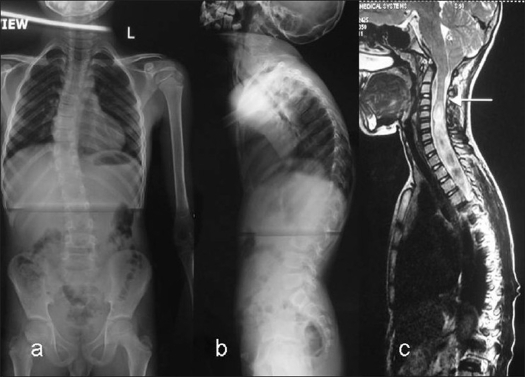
A 13 year old girl presented with a recent onset right thoracic scoliosis. Clinical examination was normal. (a, b) The whole spine X-ray showed a minimal coronal plane deformity, however there was a thoracic apical kyphosis. (c) The MRI showed a large cervico-thoracic syrinx
